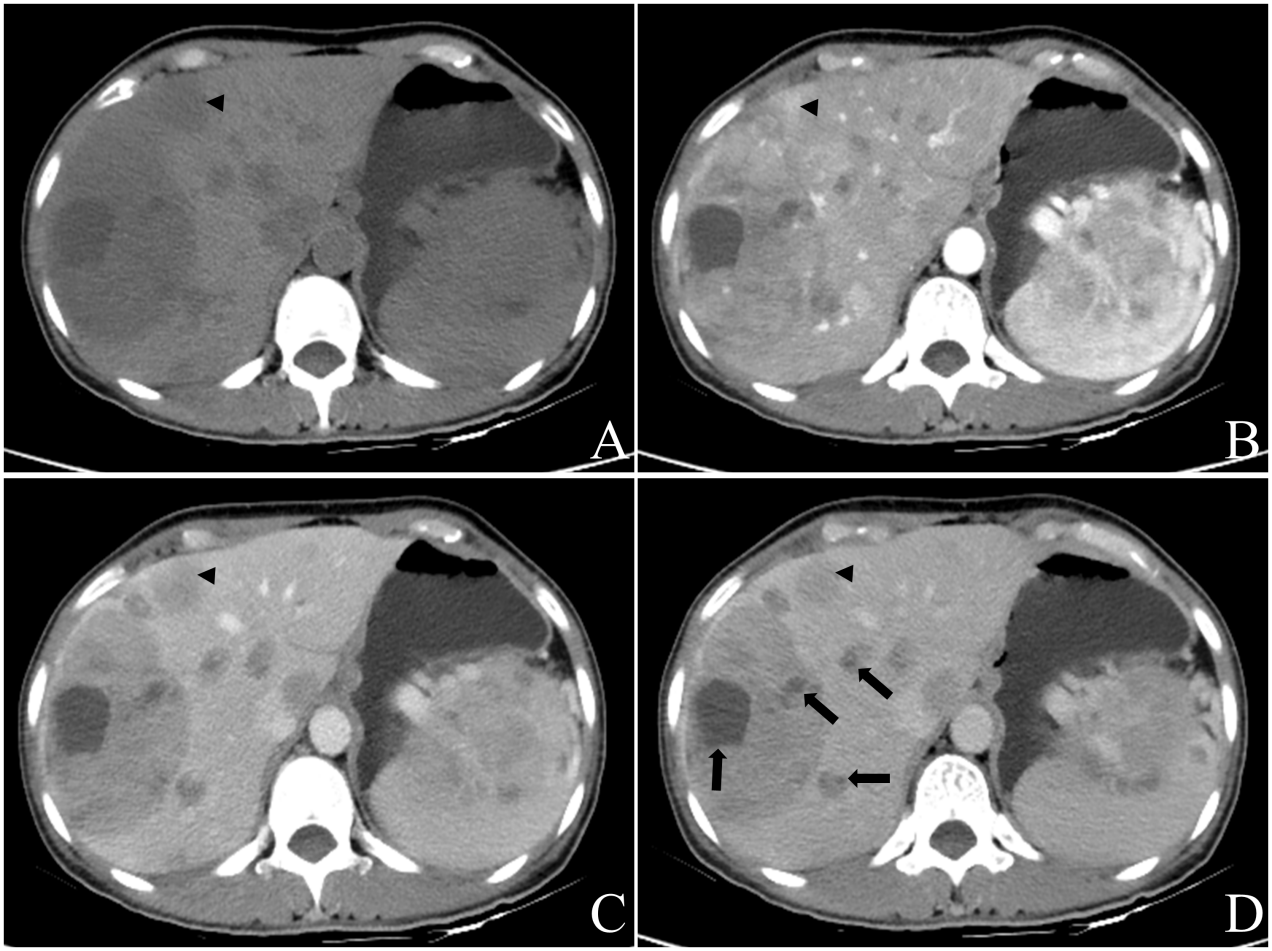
Female, aged 49 years, incidentally discovered multiple liver masses. The levels of CEA were measured at 10.53ng/ml, PIVKA at 56mAU/ml, CA199 at 237.15U/ml, and NSE levels were within the normal range. Based on contrast-enhanced CT in July 2022, the preliminary diagnosis included: (1) gastric malignant stromal tumor with multiple hepatic metastases; (2) possibility of primary liver tumors not excluded due to the presence of a large mass in the right lobe. Multiple round-like masses were observed throughout the liver, with the largest lobulated mass measuring approximately 102.7mm x 71.9mm in cross-section located in S7 and S8 segments. The contrast-enhanced scan revealed irregular annular arterial phase hyper-enhancement and peripheral “washout” in venous portal phase and delayed phase. Some smaller lesions exhibited whole-tumor enhancement (A-D), black triangle). Additionally, multiple cystic foci were identified mainly in the periphery of the lesion as well as smaller lesion (D), black arrow). Biopsy results confirmed a neuroendocrine tumor (G2) for this liver mass, and the patient remains alive with no evidence of extrahepatic NENs. The pathology report for another operation involving caudal pancreatic space occupying lesion showed solid pseudopapillary tumor of the pancreas.
